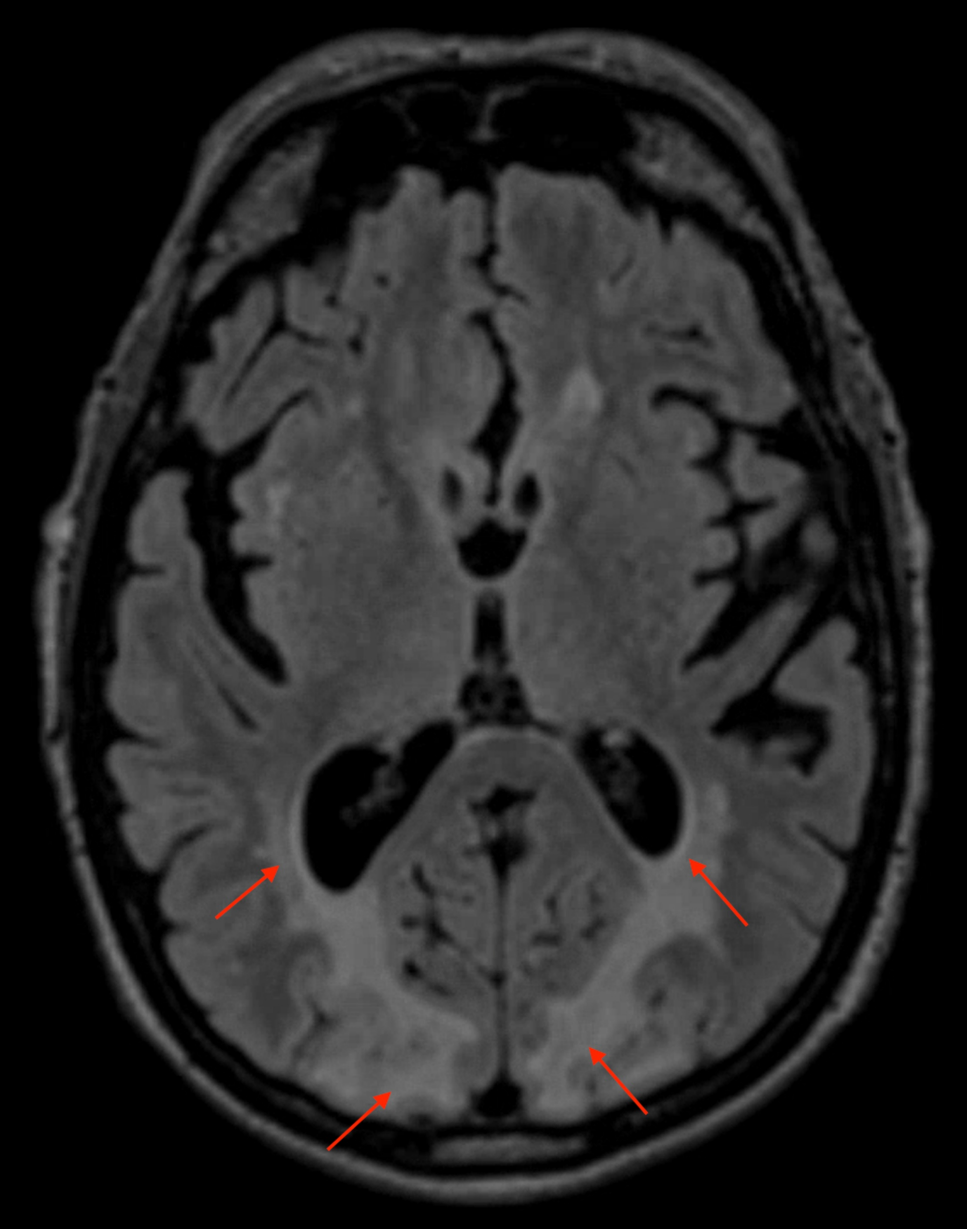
Fluid-attenuated inversion recovery (FLAIR) sequence MRI brain shows extensive oedema of occipital lobes
